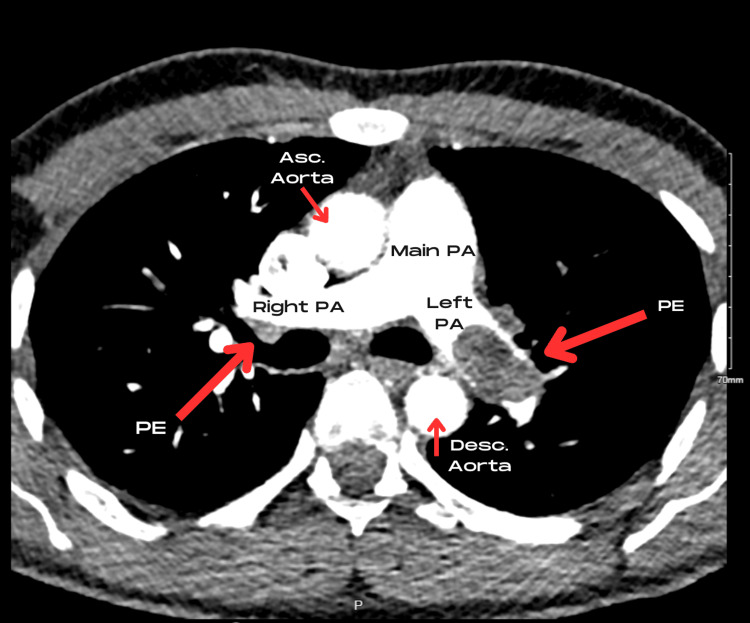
Bilateral pulmonary emboli visualized on CT pulmonary angiogram. PE: Pulmonary Embolism; PA: Pulmonary Artery; Asc. Aorta: Ascending Aorta; Desc. Aorta: Descending Aorta.
